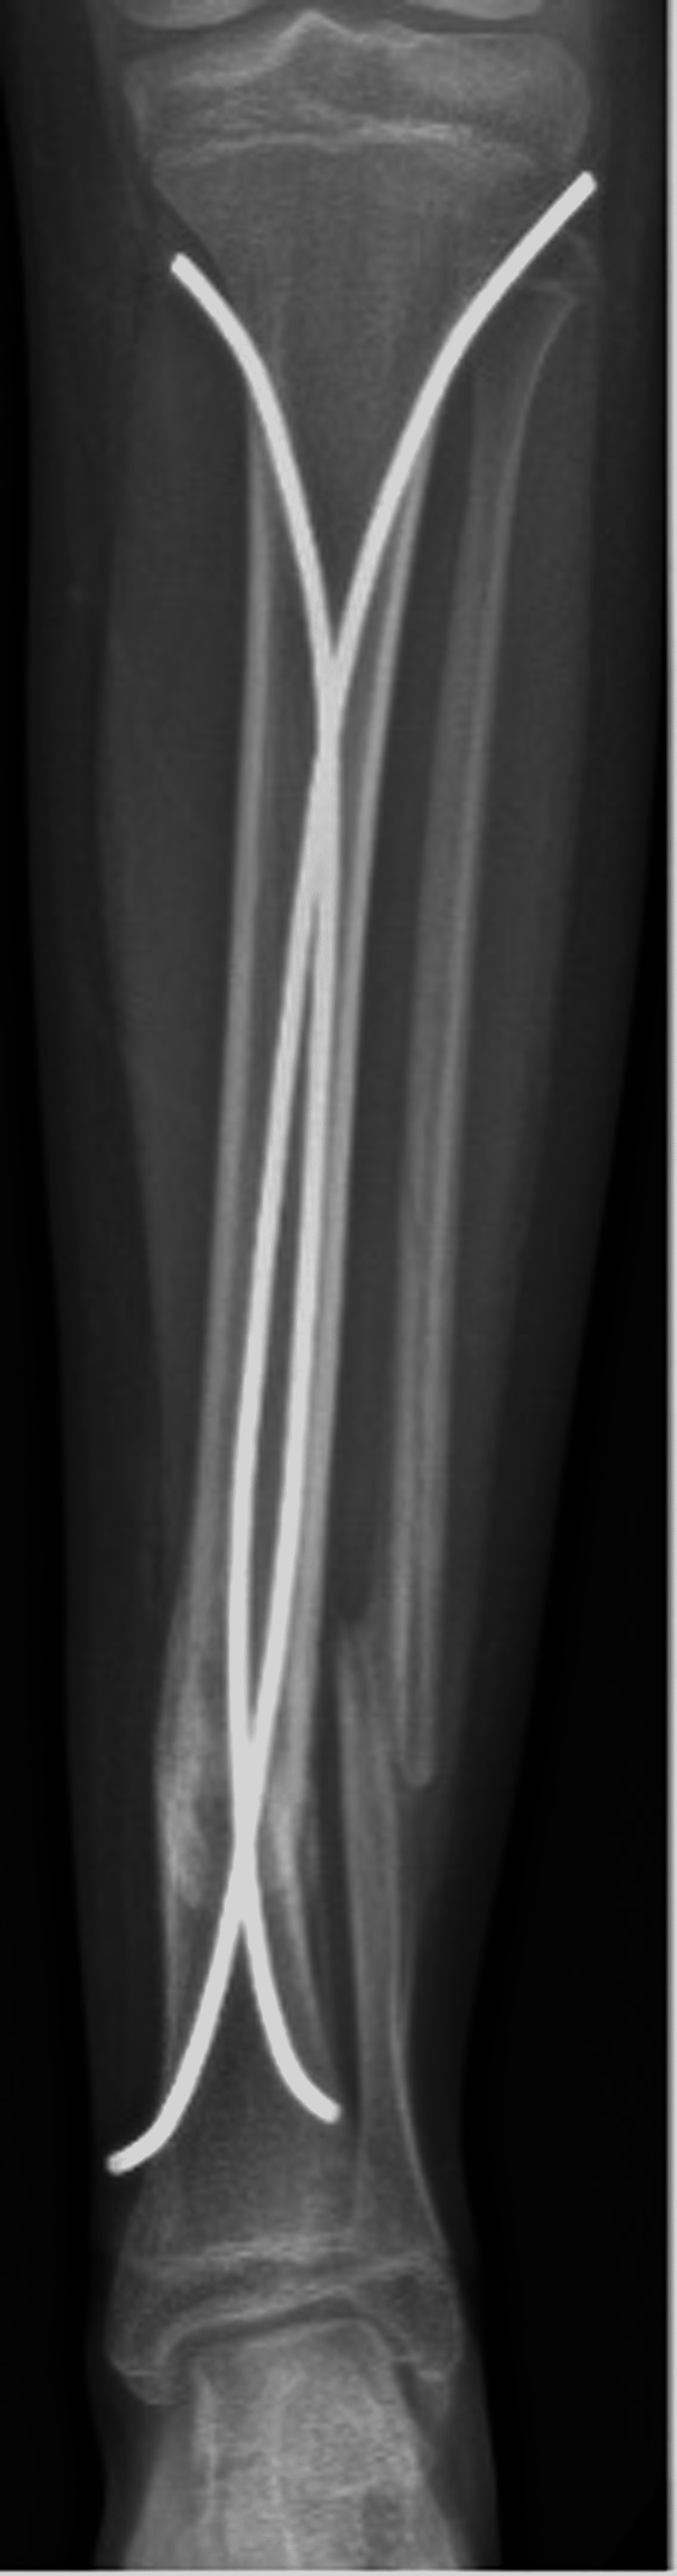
Three-month post-operative anterior-posterior tibia-fibula radiographs of flexible nailing of tibial shaft.
